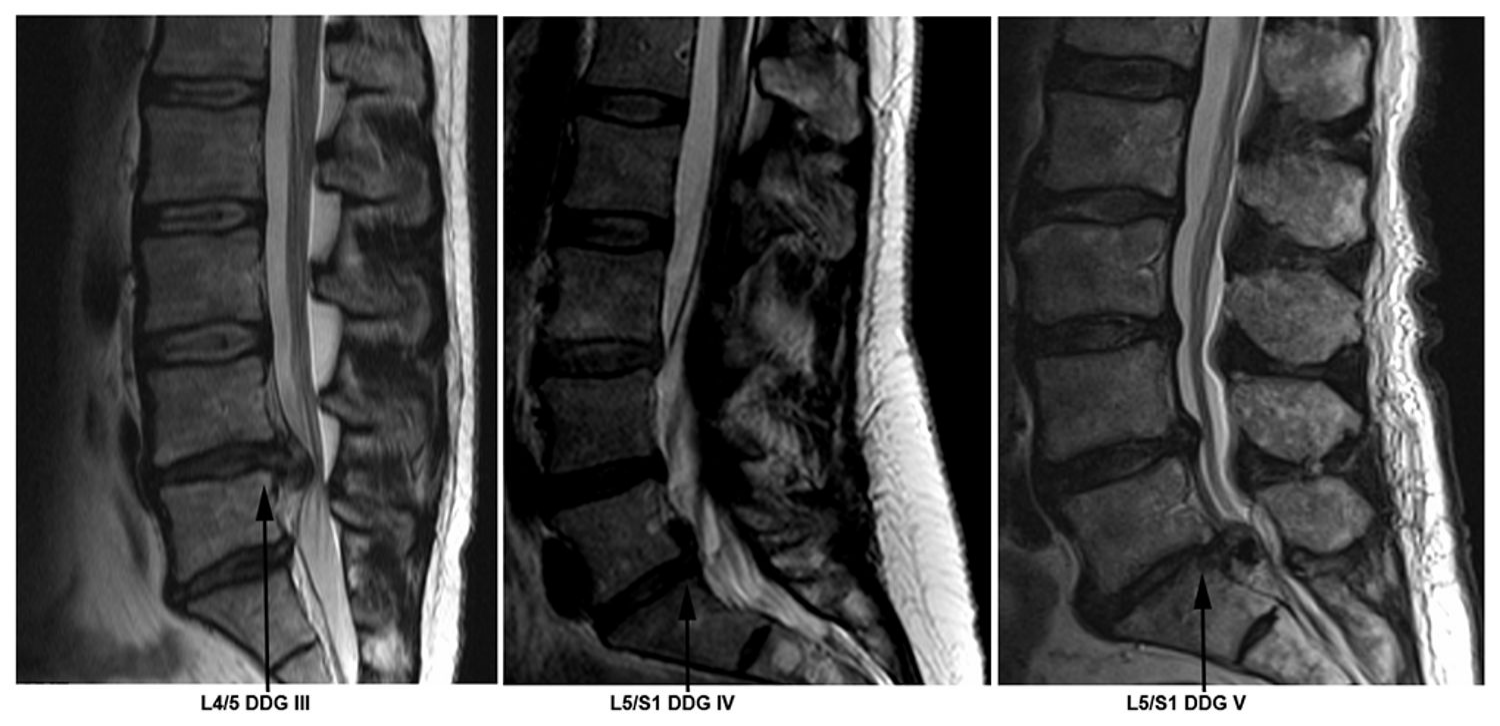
MRI showing representative images of disc degeneration grades (DDG) III, IV and V with lumbar disc herniation.
